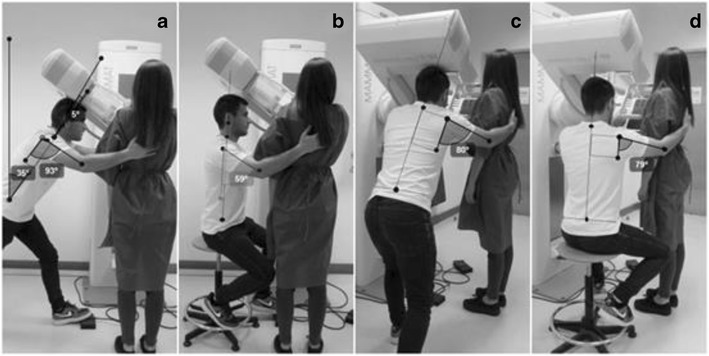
MLO breast positioning: a and c radiographer postures without intervention; b and d alternative postures when the radiographer and patient have identical statures
